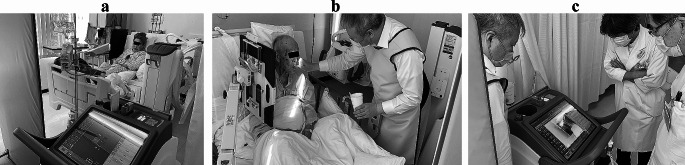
Bedside diagnosis of silent aspiration using mobile dynamic digital radiography. (a) The tube is turned toward the window side where no people are present, and the detector is affixed to a holder at the side of the patient. (b) Barium swallow is conducted using a 5-ml syringe under lateral imaging. (c) Videoclips are reproduced on the M-DDR monitor immediately after imaging to confirm whether aspiration is present
